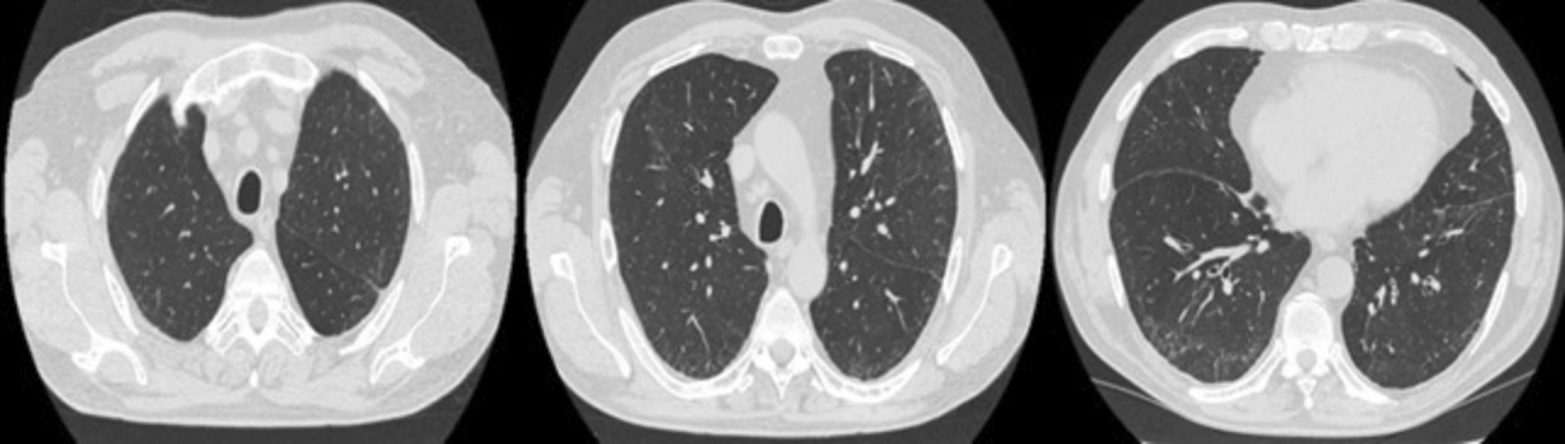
Chest CT performed 6 months after initiating mepolizumab. Near complete resolution of interstitial lung abnormalities 6 months after initiating mepolizumab therapy (some scars areas can be seen in the right lower lobe).
